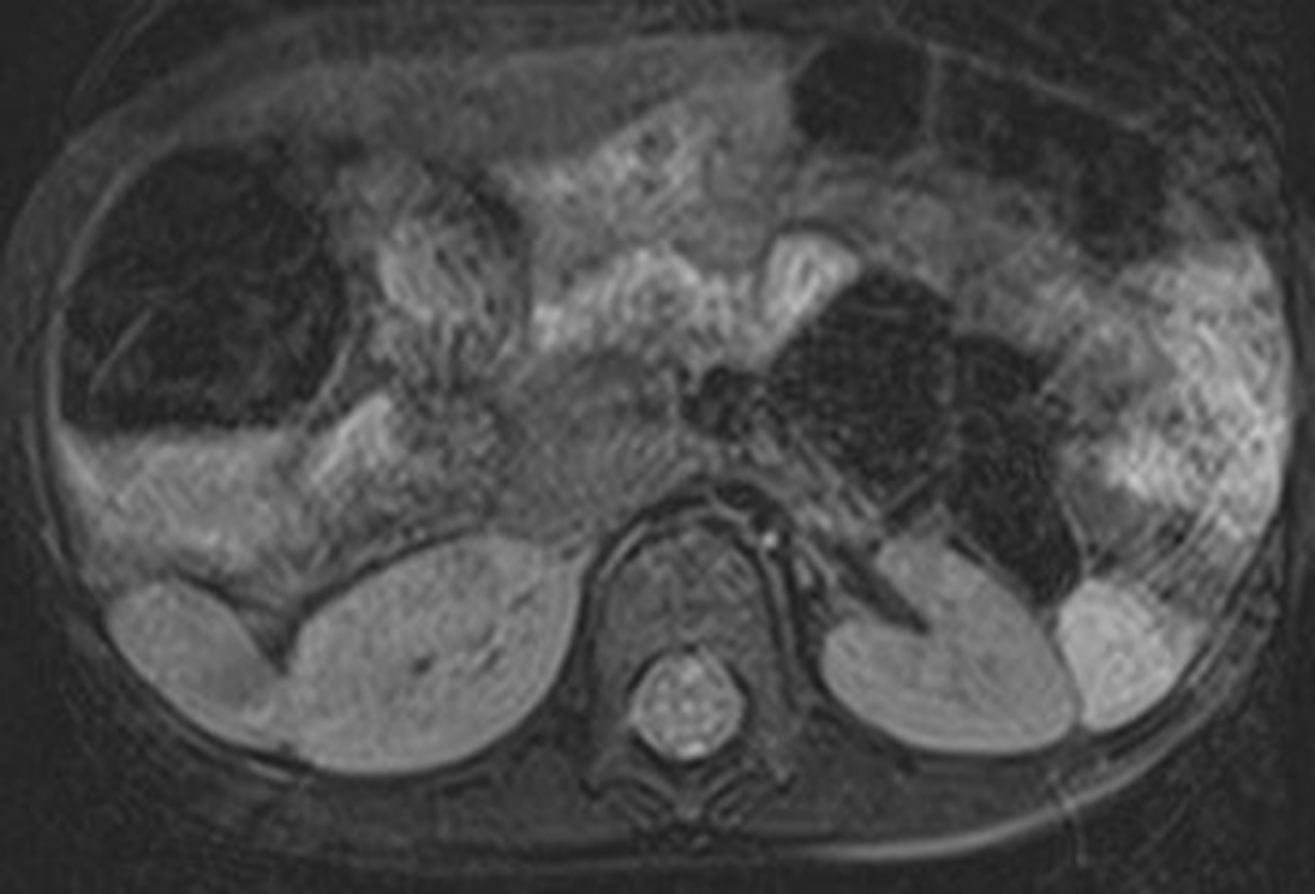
A free breathing MRI Axial view showing further the relationship between the right kidney and a spleen.
